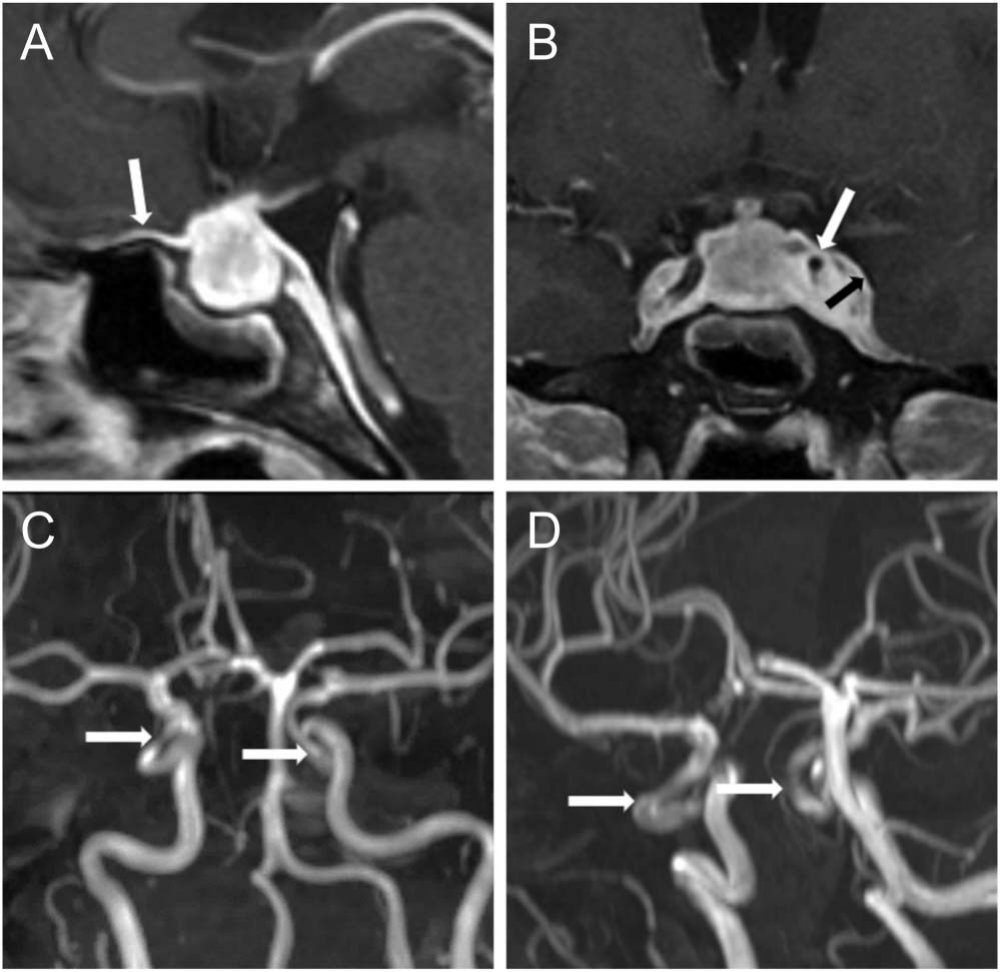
Case 2. (A) Sagittal T1-weighted MRI post-contrast at initial presentation shows intrasellar hypophysitis with suprasellar extension. Strong dural enhancement (arrow) and swelling of the sphenoid sinus mucosa are found. (B) Coronal T1-weighted MRI post-contrast shows bilateral CS infiltration with bulging of the CS towards the temporal lobe (black arrow) and narrowing of the left ICA (white arrow). (C) 3D TOF MRA confirms bilateral constriction of the ICA within the CS (arrows). (D) Follow-up 3D TOF MRA shows regression of ICA constriction with normal ICA diameters.
